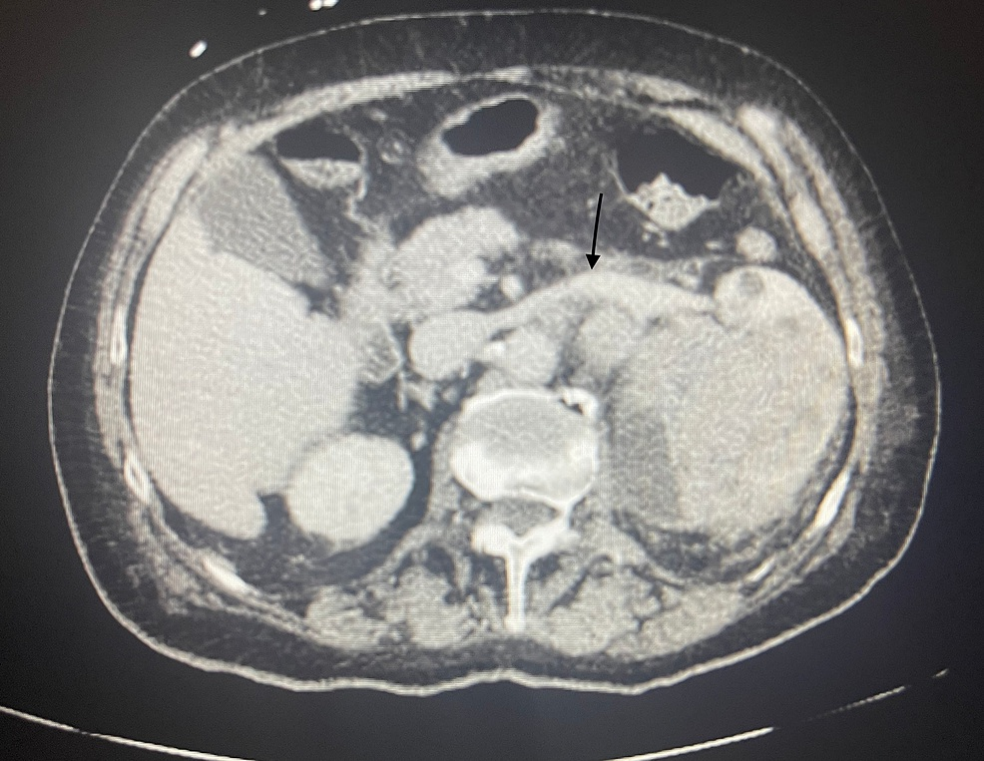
Computed tomography demonstrating the large heterogeneous solid renal mass on the left measuring 9.7 x 7.8 x 8.6 cm with extension through Gerota’s fascia and metastases to regional lymph nodes with no renal vein involvement (arrow) or distant metastatic foci noted
